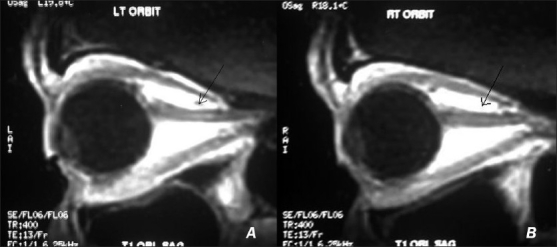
Contrast enhanced MRI showing left (A) and right (B) optic nerves at index event
